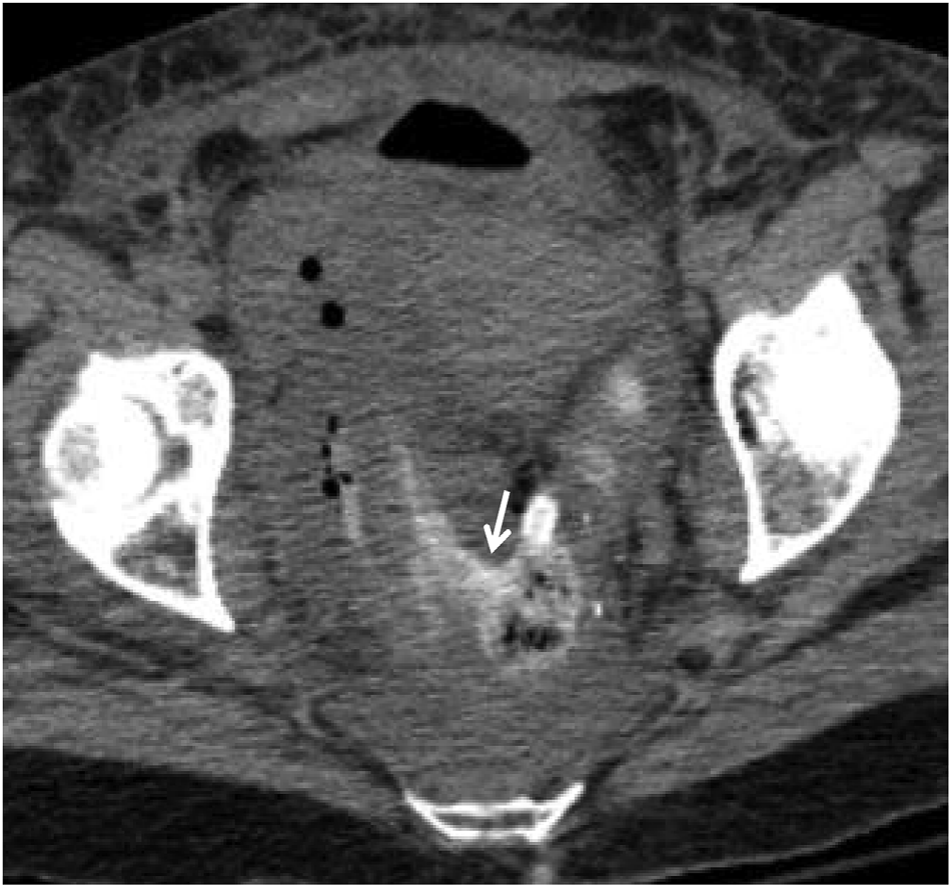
CT axial image: Retrograde filled rectum with water soluble contrast enema. A predominately gas-fluid cavity is shown in the pelvis adjacent to the right rectal wall, communicating with the rectal lumen, consistent with a giant rectal diverticulum (arrow depicts the neck of the diverticulum, connecting rectum with the diverticular cavity).
